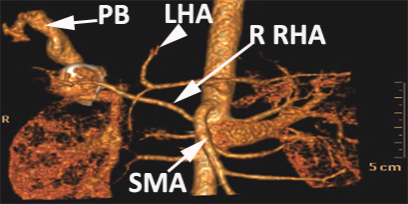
CT angiography. PB – portal branch. SMA – superior mesenteric artery. R RHA – replacing right hepatic artery. LHA – left hepatic artery.
